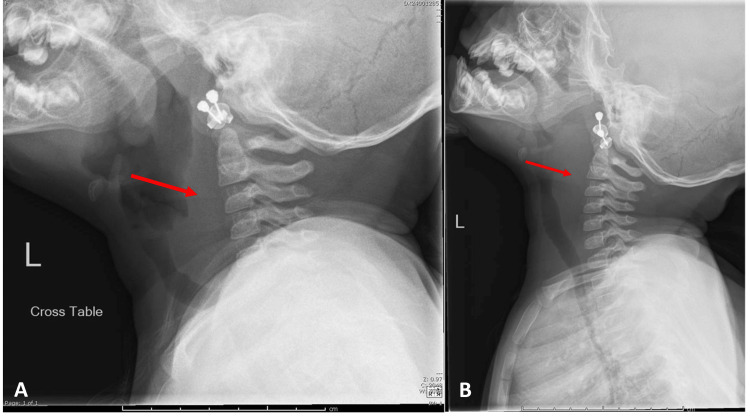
Soft tissue lateral X-rays demonstrating retropharyngeal widening (red arrows)
